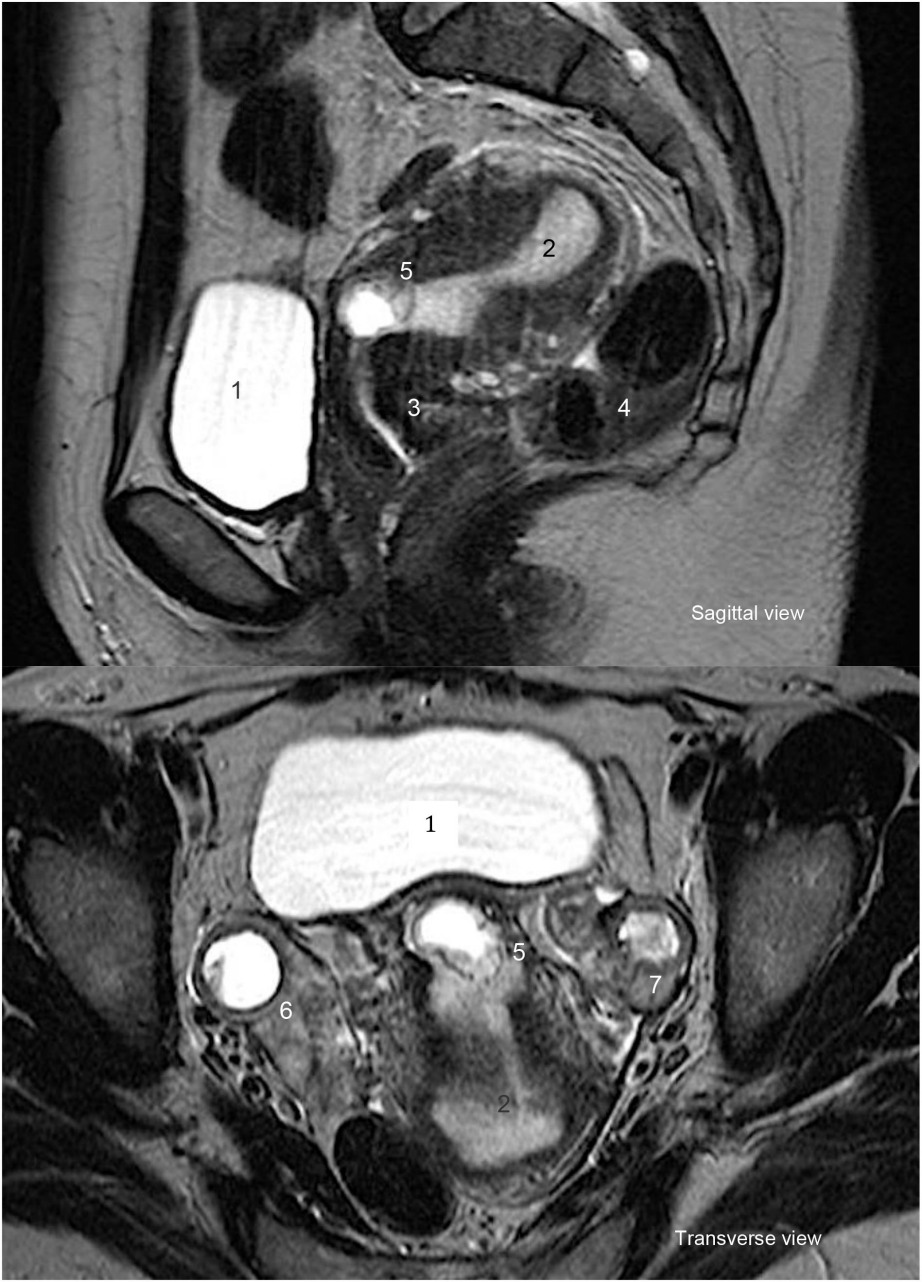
Cesarean scar pregnancy—MRI. 1. Bladder. 2. Uterine cavity. 3. Cervix. 4.Bowel. 5. Cesarean scar pregnancy. 6. Left ovary. 7. Right ovary.
